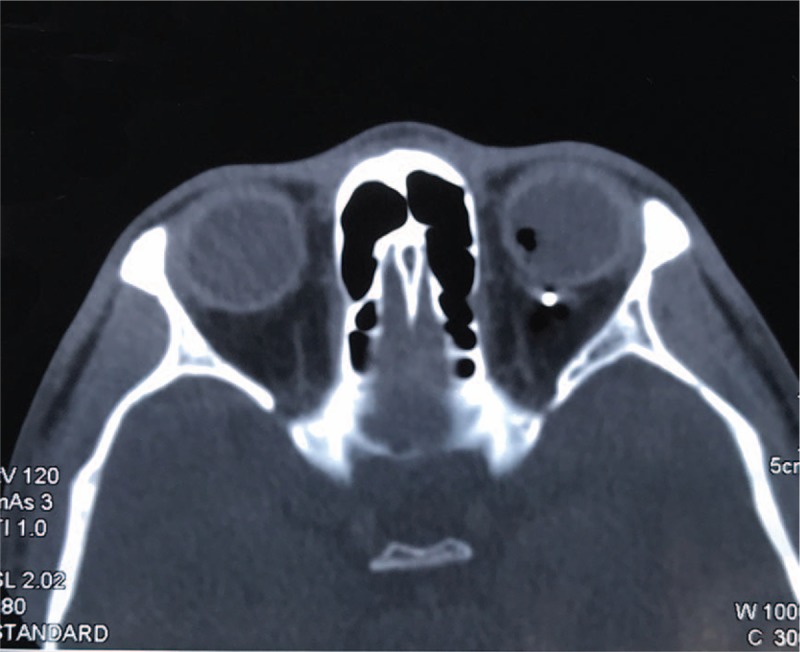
Orbital CT of a 28-year-old man with a double-penetrating ocular injury. Orbital CT scan revealed a high-density foreign body in the posterior region of the left retrobulbar space.
